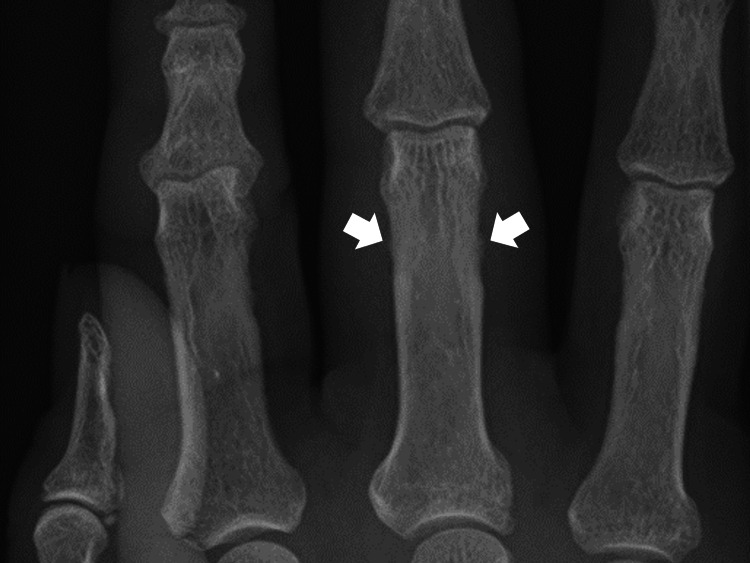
X-ray of the hand showing osteophytes at the site of tendon attachment around the proximal interphalangeal joint of the right middle finger (white arrows).
